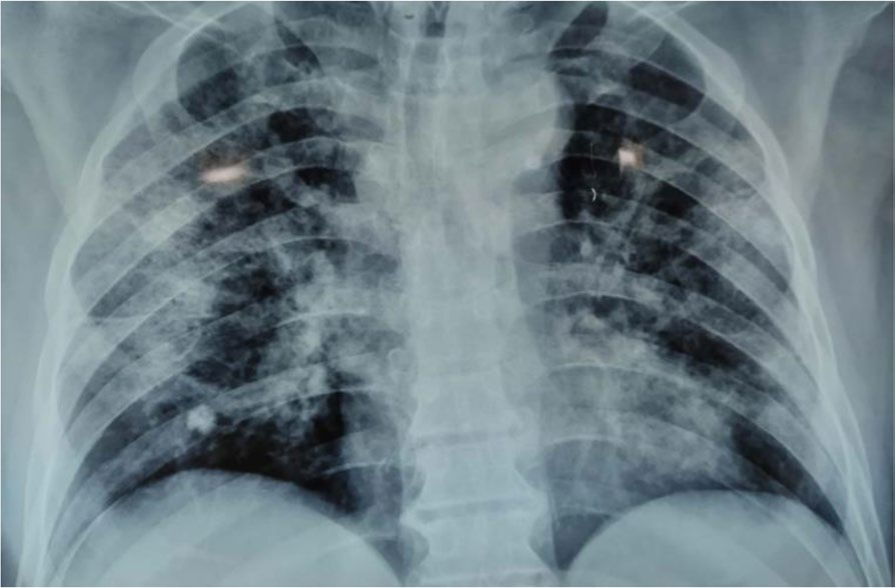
Initial chext X-ray at presentation to the ER which shows B/L diffuse patchy infiltrates
